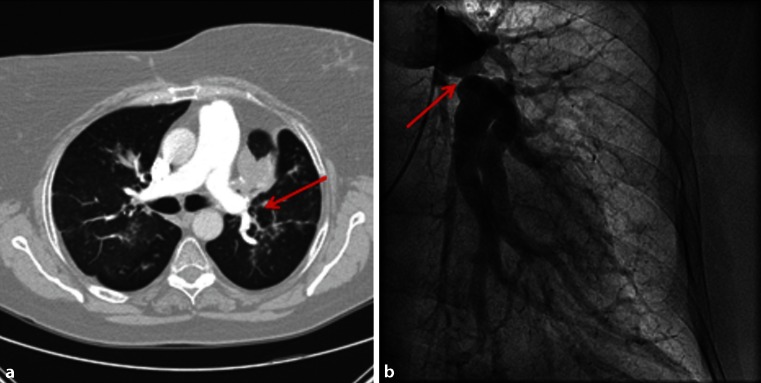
Compression of the pulmonary artery. A case of a 60-year-old female with stage IV sarcoidosis, diagnosed with PH with a mean PAP of 63 mmHg, due to compression of the pulmonary artery. a Compression on chest CT; b Pulmonary angiogram of the left lobe
